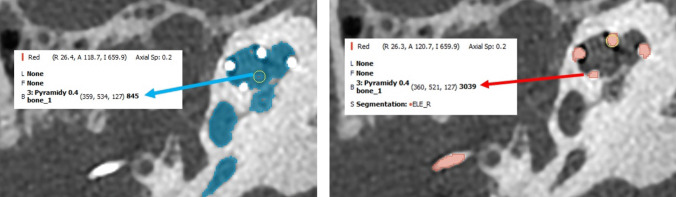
Example of greyscale thresholding when using CT images: membranous labyrinth (dark= labyrinth; 0 and 1000); electrode (bright= bone and metal; > 2500)
